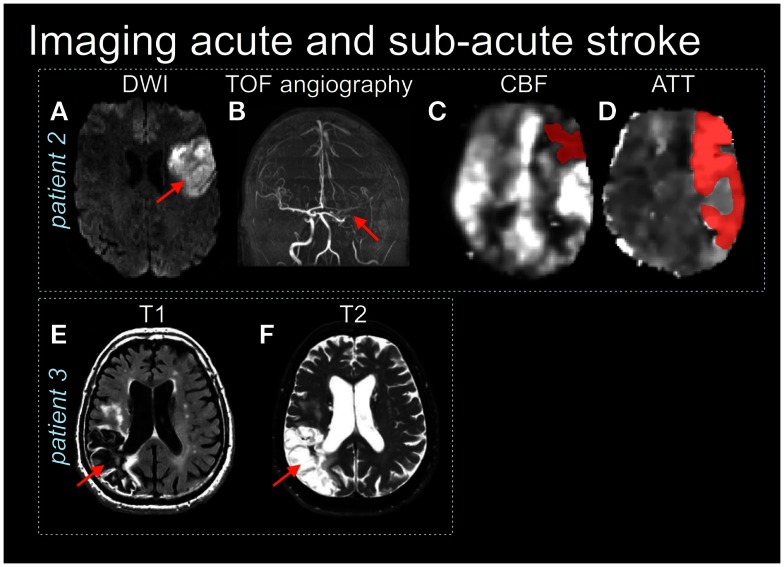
Patient 2 is an acute stroke with mild impairment (NIHSS = 1) yet the diffusion-weighted image (DWI) (A) shows restricted diffusion in the left frontal lobe (red arrow) and a large vessel obstruction on the × (TOF) angiography (B). (C,D) Arterial spin labeling images show reduced cerebral blood flow (CBF) and prolonged arterial transit time (ATT) (shaded in dark red) (Based on MacIntosh et al., 2010). Patient 3 shows the right parietal lobe stroke lesion on T1 (E) and T2-weighted (F) images.
